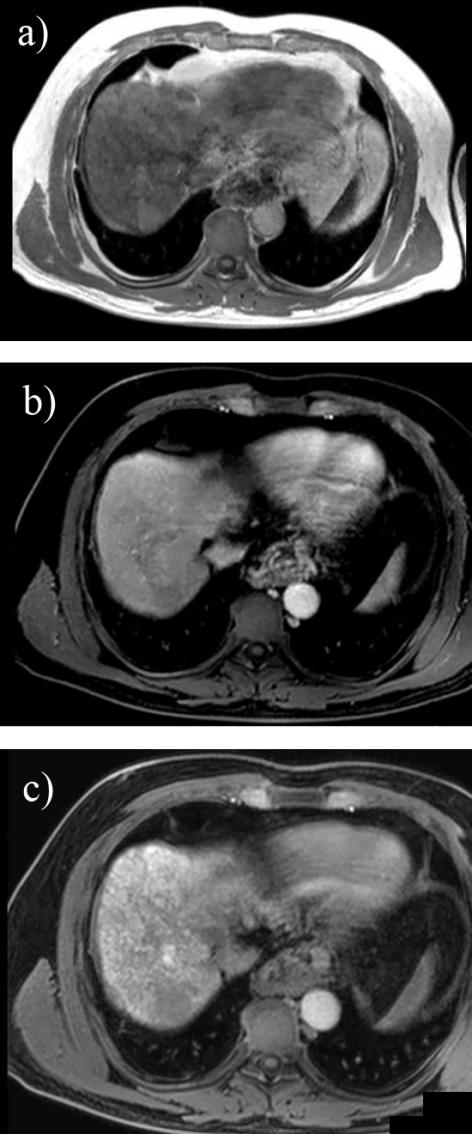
3T MRI in a cirrhotic patient using Gd-EOB-DTPA and revealing a small nodule in segment VII with high signal in axial T1 in phase (a), low signal in dynamic postcontrast (b) and at 30 minutes in hepatobilliary phase (c) suggesting the diagnosis of HCC
